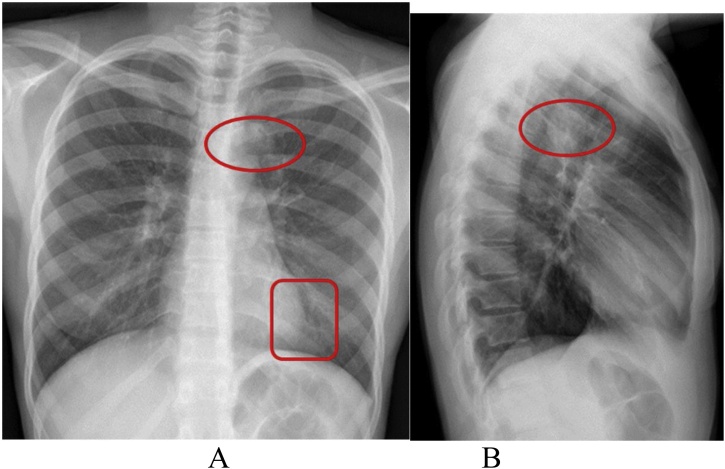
A, B: PA and lateral chest X-Ray: Left upper lobe apicoposterior segment opacity obscuring aortic knob (shown by ). Left lower zone opacity obscuring left cardiac border (shown by ).
